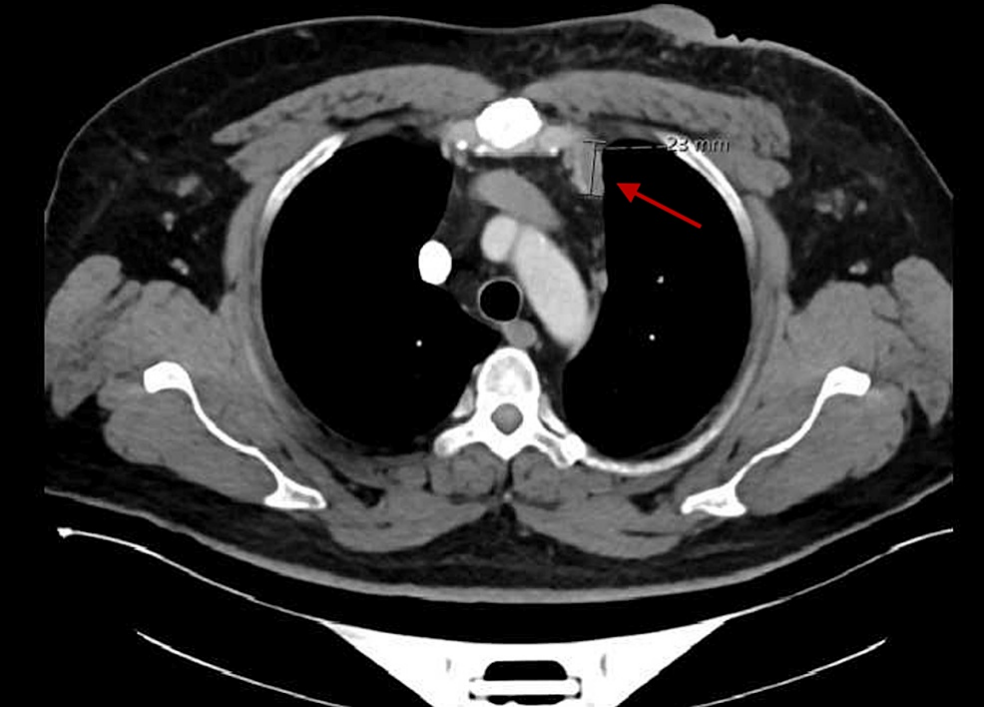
CT thorax showing an enlarged lymph node at the manubrium sternal junction
